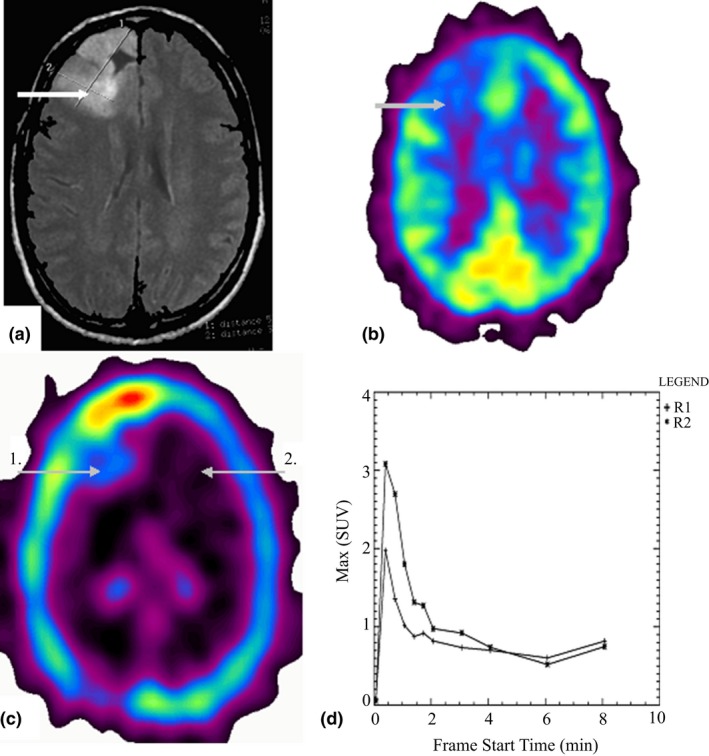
(a‐d) Male patient i. Diagnosis: astrocytoma of low malignant potential (Gr II). (a) MRI T1 WI image demonstrates a space‐occupying lesions of the anterior convexital parts of the right frontal lobe. (b) ^18^F‐FDG PET image demonstrates that the lesion is partially visualized due to its white matter location as well as to the perifocal edema, and the level of the glucose metabolism is low (UI = 0.6). (c) ^82^Rb‐chloride PET image demonstrates the isovascular focus with low radiopharmaceutical uptake (UI = 1.2) in the projection of the lesion continued growth (position 1). Contralateral brain cortex is shown in position 2. (d) Activity/Time curve obtained in ^82^Rb‐chloride PET shows that the lesion (1) has moderate vascularization degree (VD = 1.6). The fact that radiopharmaceutical uptake is weak and low and radiopharmaceutical does not linger in the tissue phase indirectly points at the low malignant potential of the lesion. The activity/time curve (2) over contralateral cortex is shown for comparison
